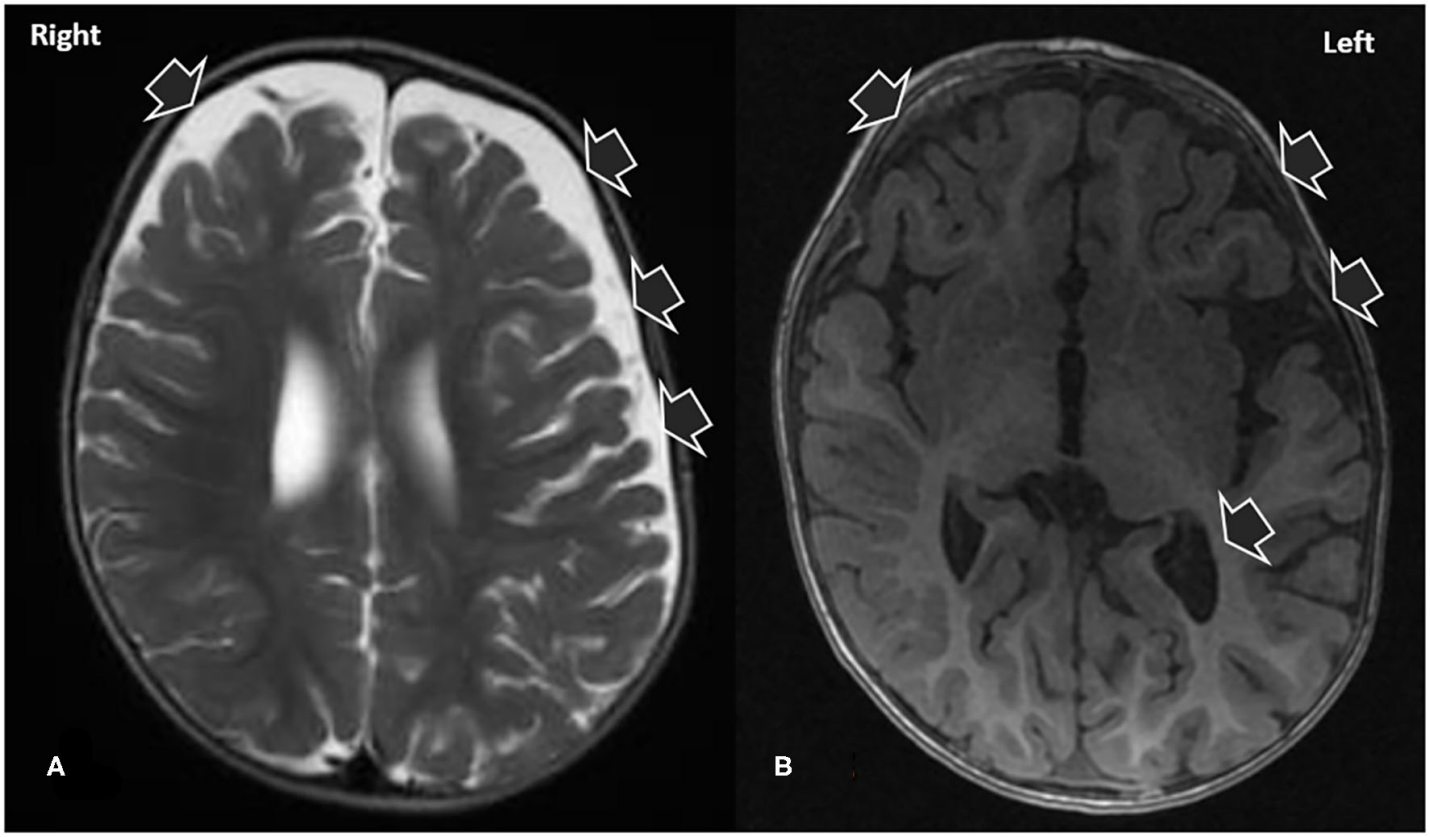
T2 (A) and T1 (B) magnetic resonance images demonstrating signs of craniofacial disproportionality and signs consistent with macrocrania. Note that the patient had an overall normal morphology, excepting the prominence of the ventricular system and CSF-filled spaces in the left cerebral convexity.
